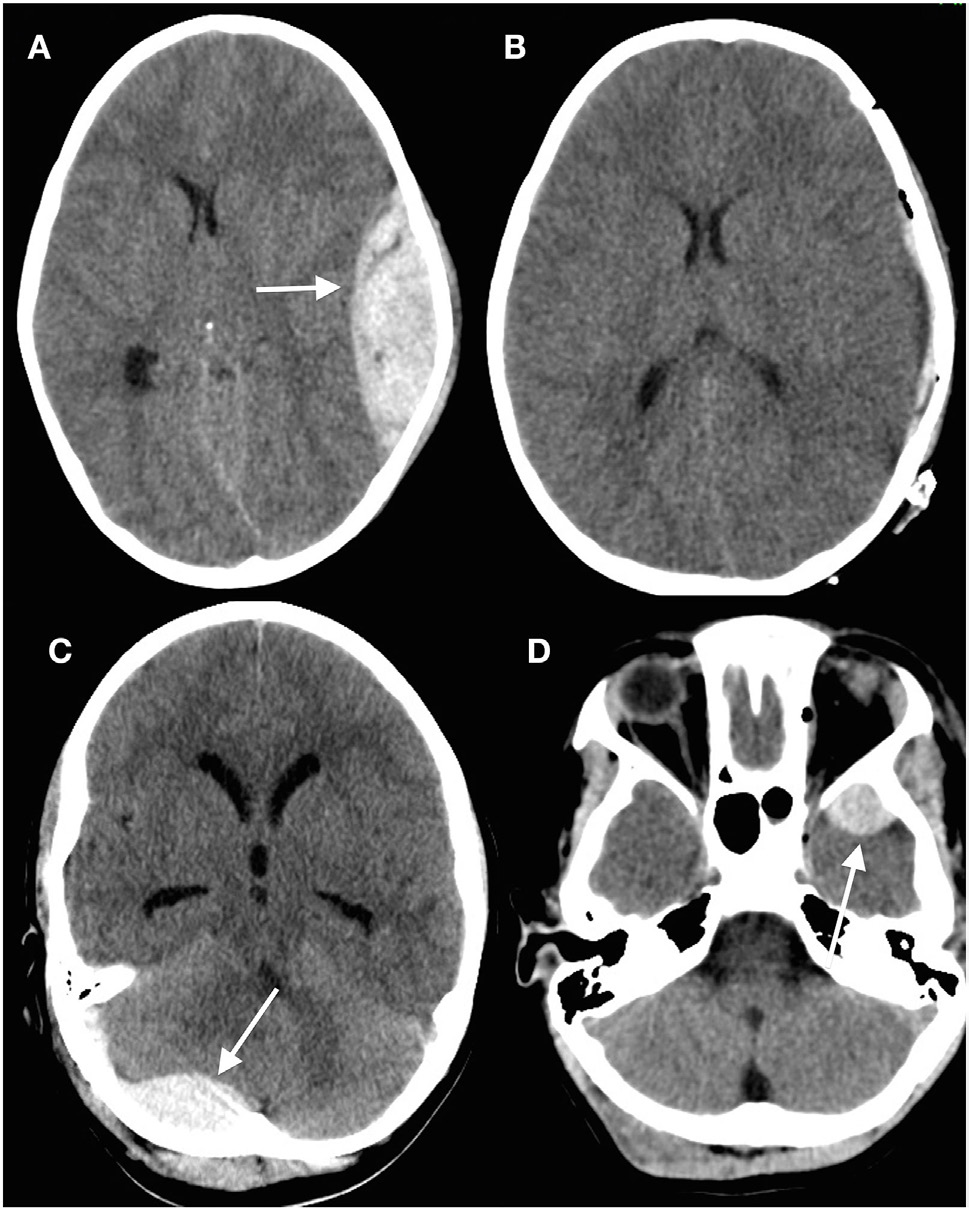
Epidural hematomas occur in a variety of locations. (A) Head computed tomography (CT) showing a typical convexity epidural hematoma in a child; (B) evacuated hematoma in the same patient; (C) posterior fossa epidural hematoma (arrowed) underlying a suboccipital fracture; (D) epidural hematoma anterior to the left temporal tip (arrowed) (13) (modified).
